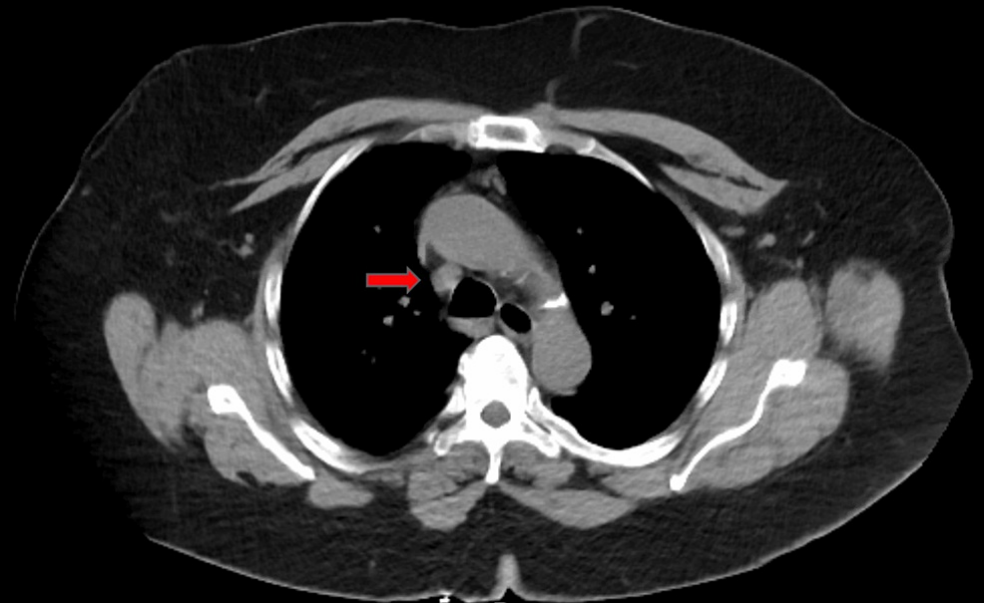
CT scan of the chest without contrast showing narrowing of the superior vena cava at the level of the aortic arch (red arrow).
